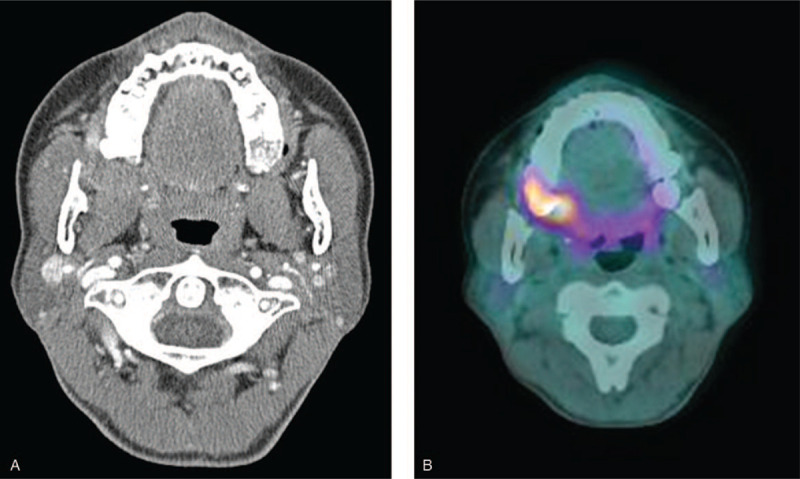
A) Spiral CT of the neck. There is a 2.4 × 1.6 × 2.0 cm sized enhanced mass around the right retromolar trigon. The mass is extended to the right buccal space and invades into part of the pterygoid muscle. B) Whole-body FDG PET/CT. There is FDG-avid mass lesion (SUVmas = 8.58) around the right retromolar trigon. CT = computed tomography, FDG = focal segmental glomerulosclerosis, PET = positron emission tomography.
